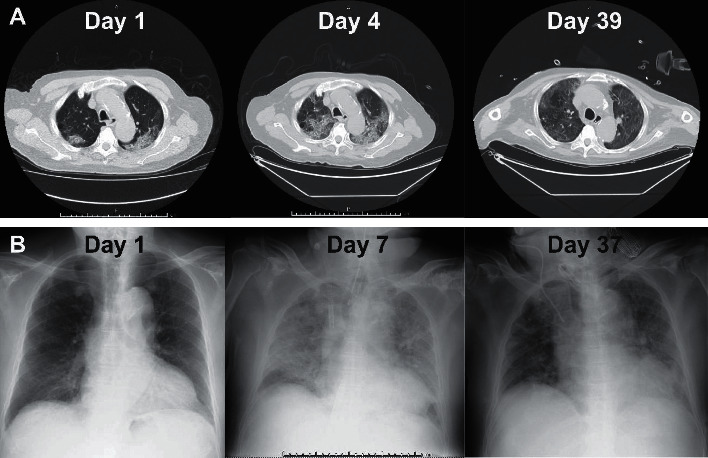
Radiological findings in the patient. (a) Transverse chest CT images on days 1, 4, and 39. Bilateral infiltrative shadows and ground-glass lesions were apparent, which deteriorated on day 4. On day 39, ground-glass lesions decreased and lung aeration improved. (b) Chest radiographs showing changes in lung opacity over time. An endotracheal tube and blood delivery cannulas for ECMO are visible in the image taken on day 7. These were removed, and a tracheostomy tube is visible in the image taken on day 37.
